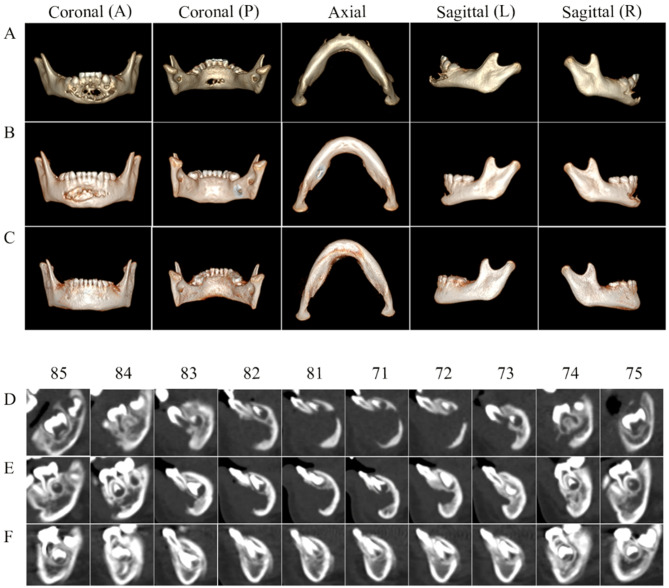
CT images of case 5 for jaw status and deciduous dentition in the mandibular. A: CT images of the osteolytic lesions in the mandibular at diagnosis from left to right - coronal (A-anterior, P-posterior), axial, and sagittal (L-left, R-right) scans. B: CT images of slow regeneration, which are 15 months later after first line treatment. C: CT images of fast regeneration and good jaw status, which are after 12 months of dabrafenib treatment. D-F: Sagittal CT images at different deciduous tooth sites from 85 to 75; the time acquiring the images corresponds to A-C, respectively
